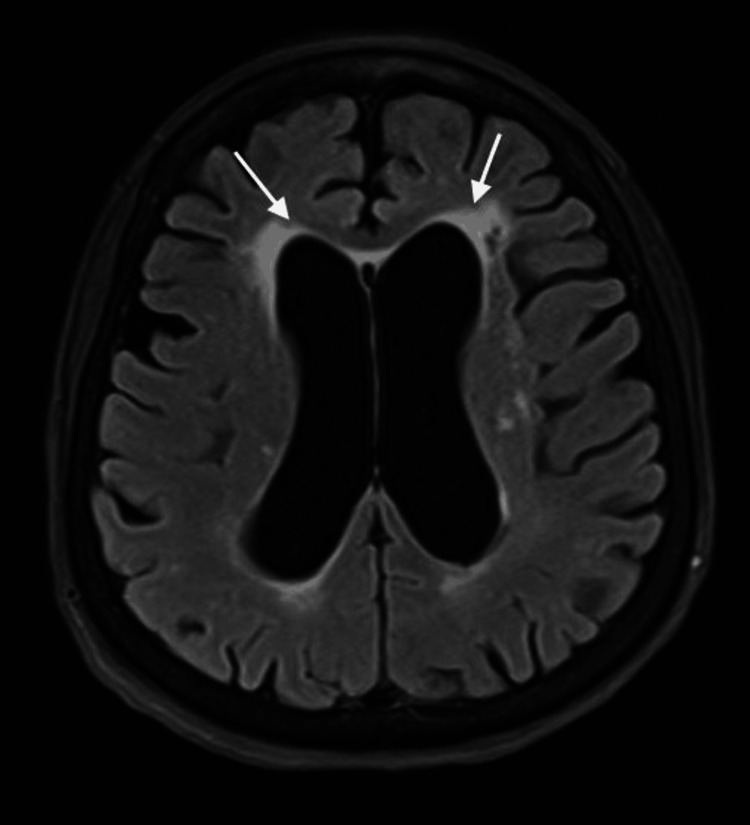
The MRI of the brain shows an axial view and a fluid-attenuated inversion recovery (FLAIR) image. Arrows pointing at periventricular hyperintensities representing small vessel disease.
